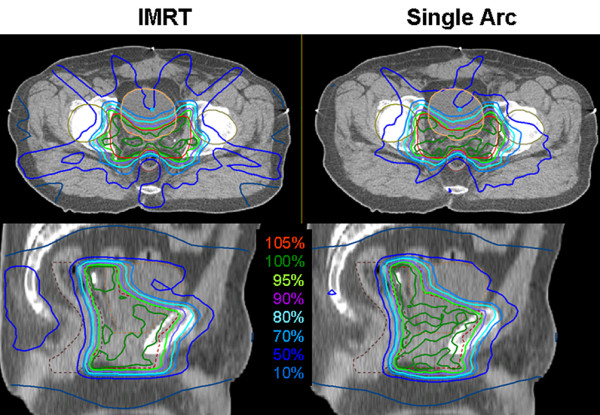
Dose distributions for prostate cancer. Comparison of dose distributions achieved with 7-field IMRT (left) and Single Arc VMAT (right) on representative transversal (top) and sagittal (bottom) CT slices. The PTV is drawn in red, the bladder in orange, the rectum in maroon, and the femoral heads in green. Isodose lines are shown in percent of the prescription dose, i.e. 60 Gy to the average of the PTV.
